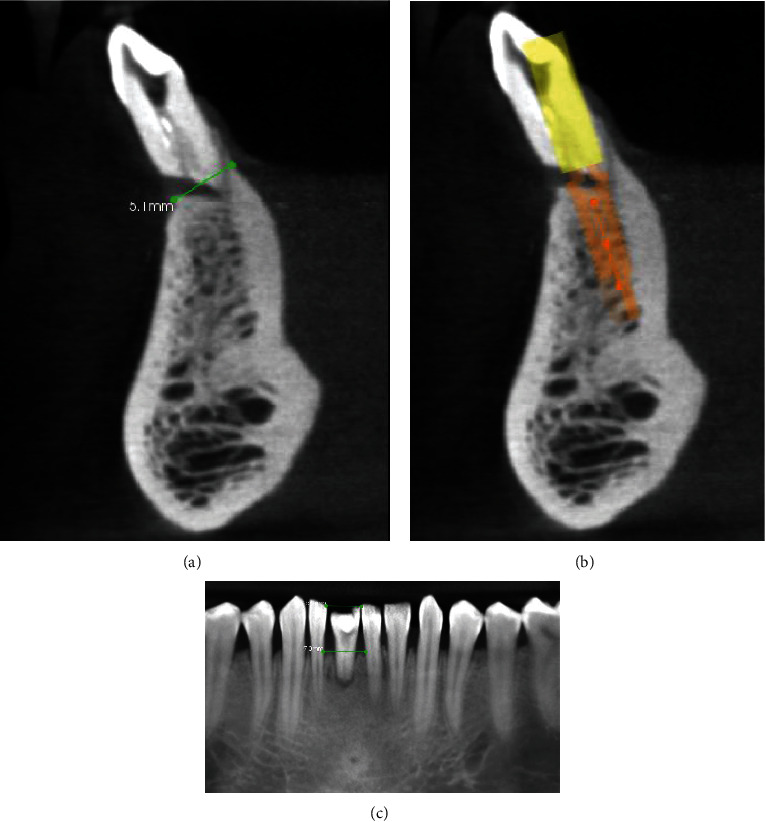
(a, b) Cone-beam computed tomography (CBCT) in sagittal section with dental implant planning with respect to 41. (c) Mesiodistal measurement at the proposed implant site.
